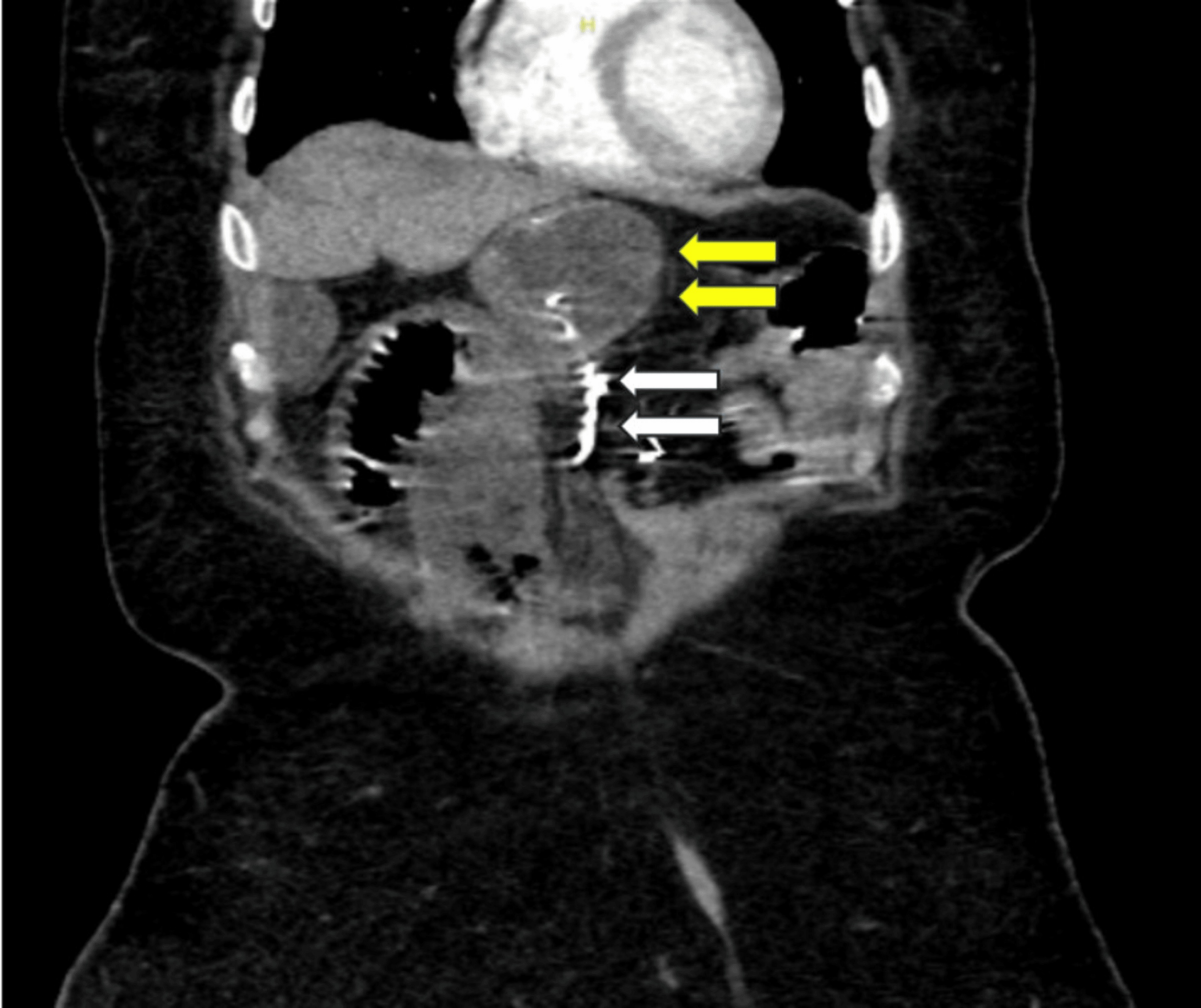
CT of the abdomen with white arrows marking the MiniMizer and yellow arrows showing a segment of an obstructed dilated small bowel loop with decreased enhancement and congested mesentery.
